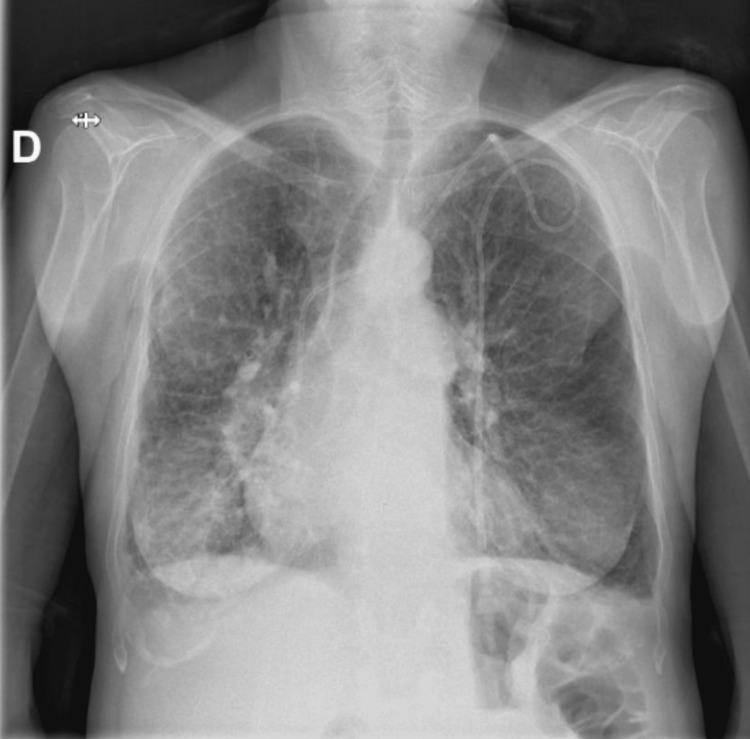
Post-operatory radiography
